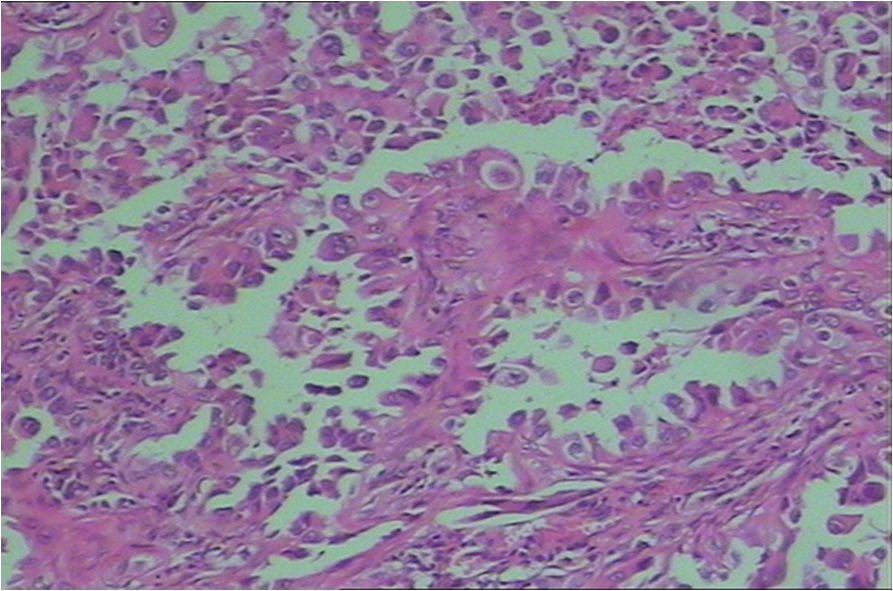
Magnetic resonance imaging (MRI) scan of the brain found dilated ventricles, diffuse white matter atrophy, and a metastatic mass about 1.5 cm in size in the right temporal lobe.
